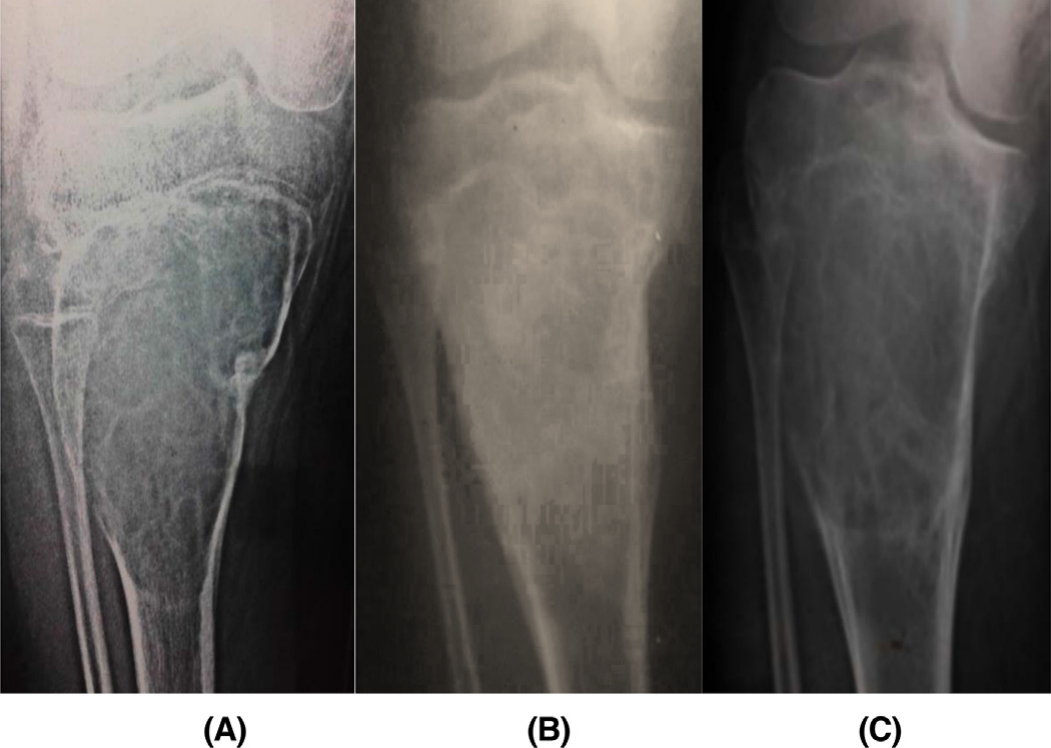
Serial radiographs of (A) 11 years old patient with ABC in the proximal tibia (case 16), (B) 3 months follow-up showed proper healing and cortical thickening, and (C) 5 months follow-up showed lysis in previously healed areas and bone expansion (signs of recurrence).
